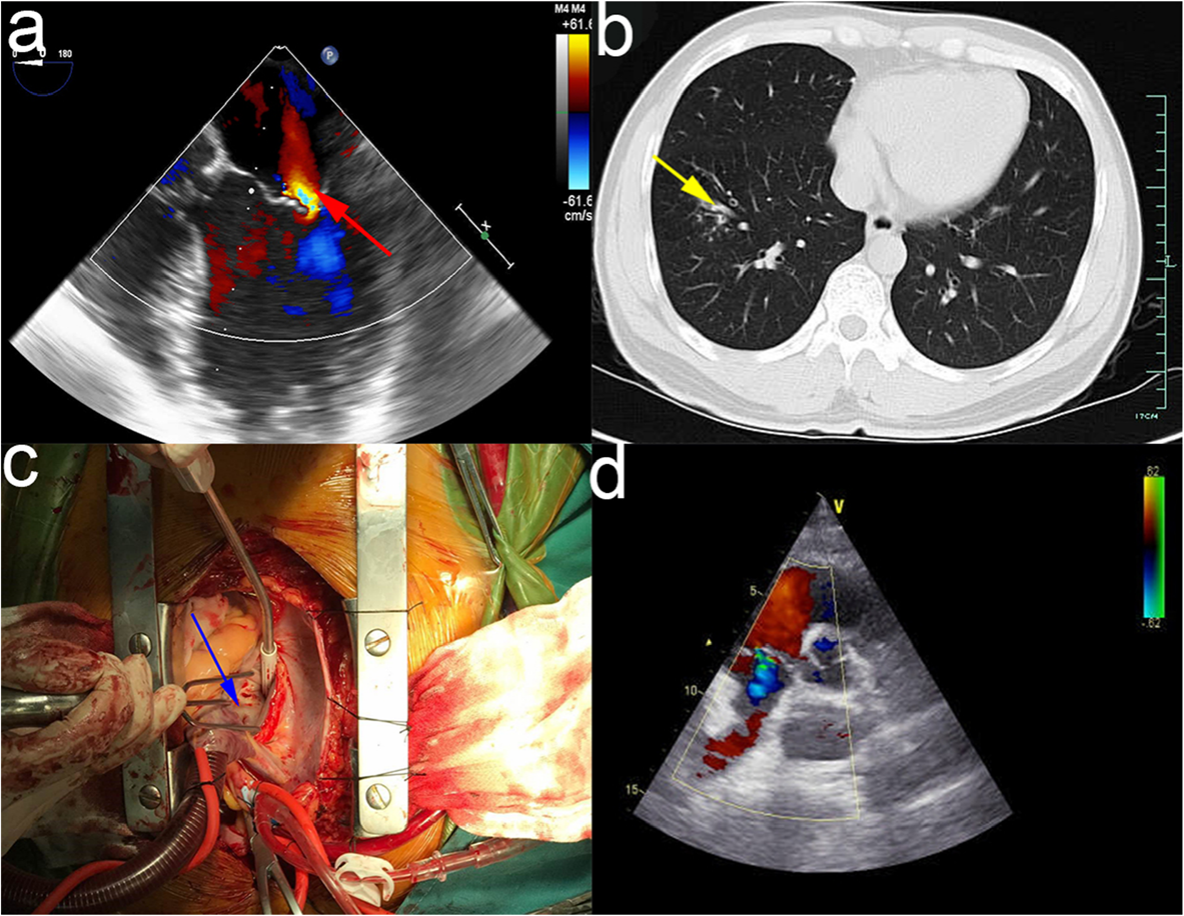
a The echocardiogram showed an abnormal perforation between the left ventricle and atrium with moderate mitral valve regurgitation. (the red arrow). b The CT scan showed there was bronchopneumonia in right lung lobe. (the yellow arrow). c The blue arrow indicated was exactly the perforation in the P2 area of mitral valve annulus, with a diameter of about 6 mm. d The post-operative echocardiography showed the mechanical valve worked well without any perivalvular leakage
